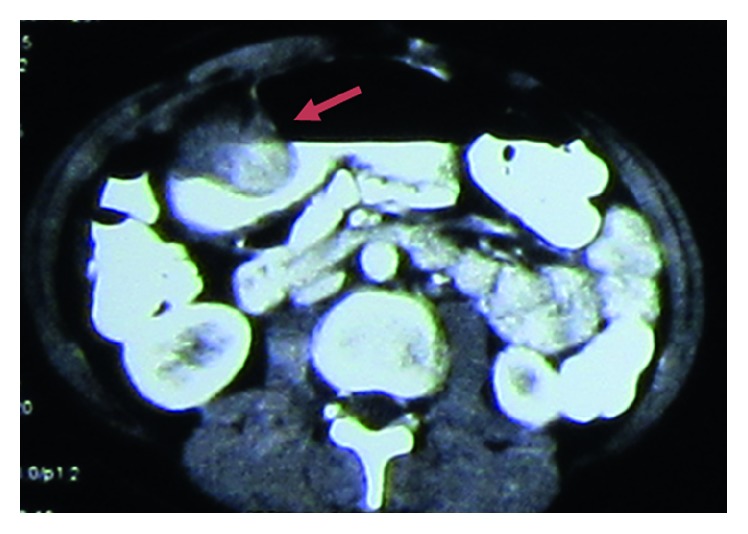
Abdominopelvic CT scan with IV and oral contrast. A 5 cm × 6 cm soft, hypodense mass along the anterior wall of the gastric antrum with subsequent pyloric stenosis and luminal obstruction with gastric dilatation in the body and fundus (the red arrow indicates the mass).
